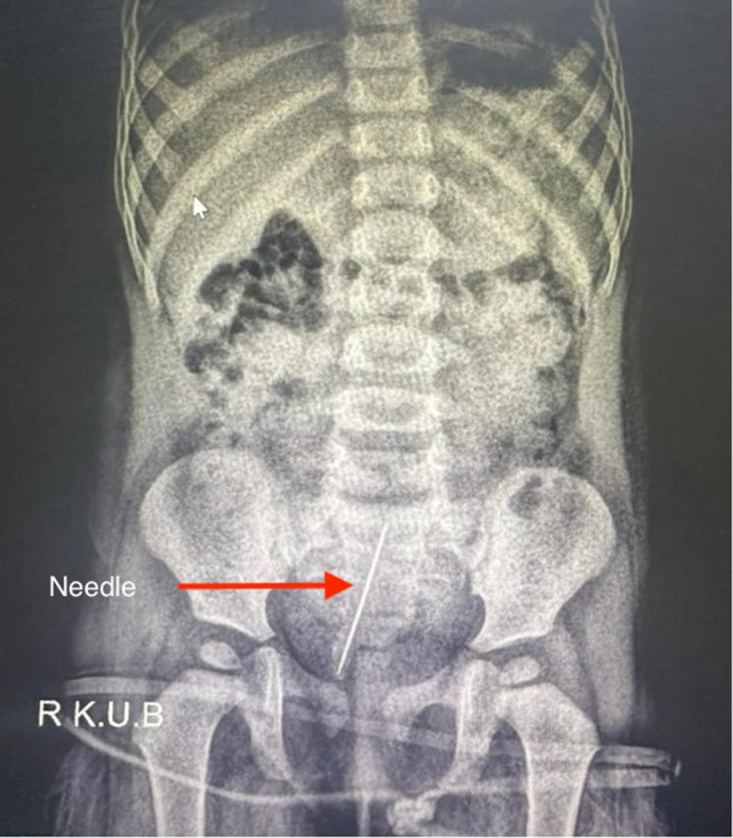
Kidney, ureter, and bladder (KUB) radiograph showing a linear radio-opaque foreign body (arrow) within the urinary bladder, consistent with a calcified sewing needle. The increased density at one end reflects mineral deposition from prolonged urine exposure.
